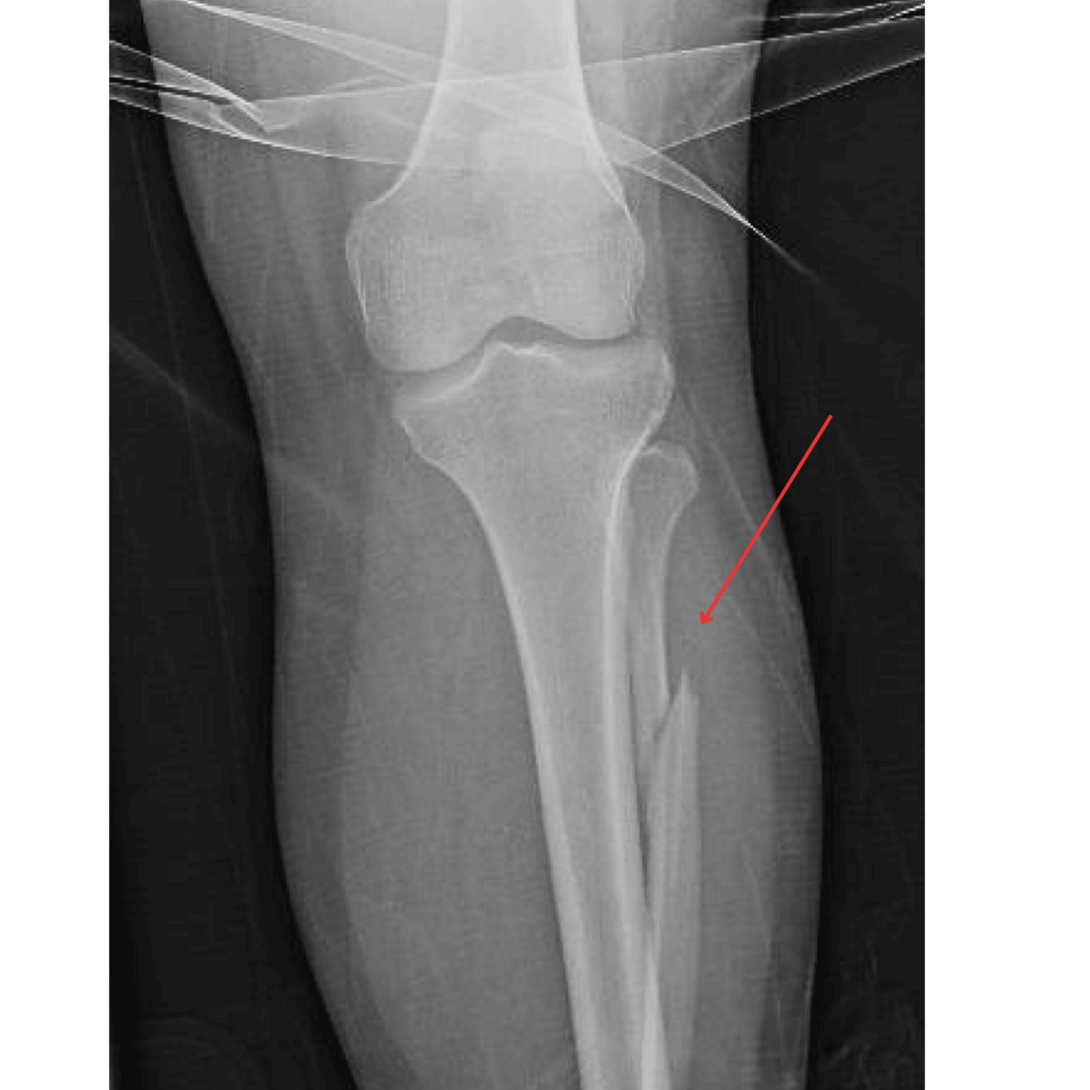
X-ray of the proximal left fibula fracture
